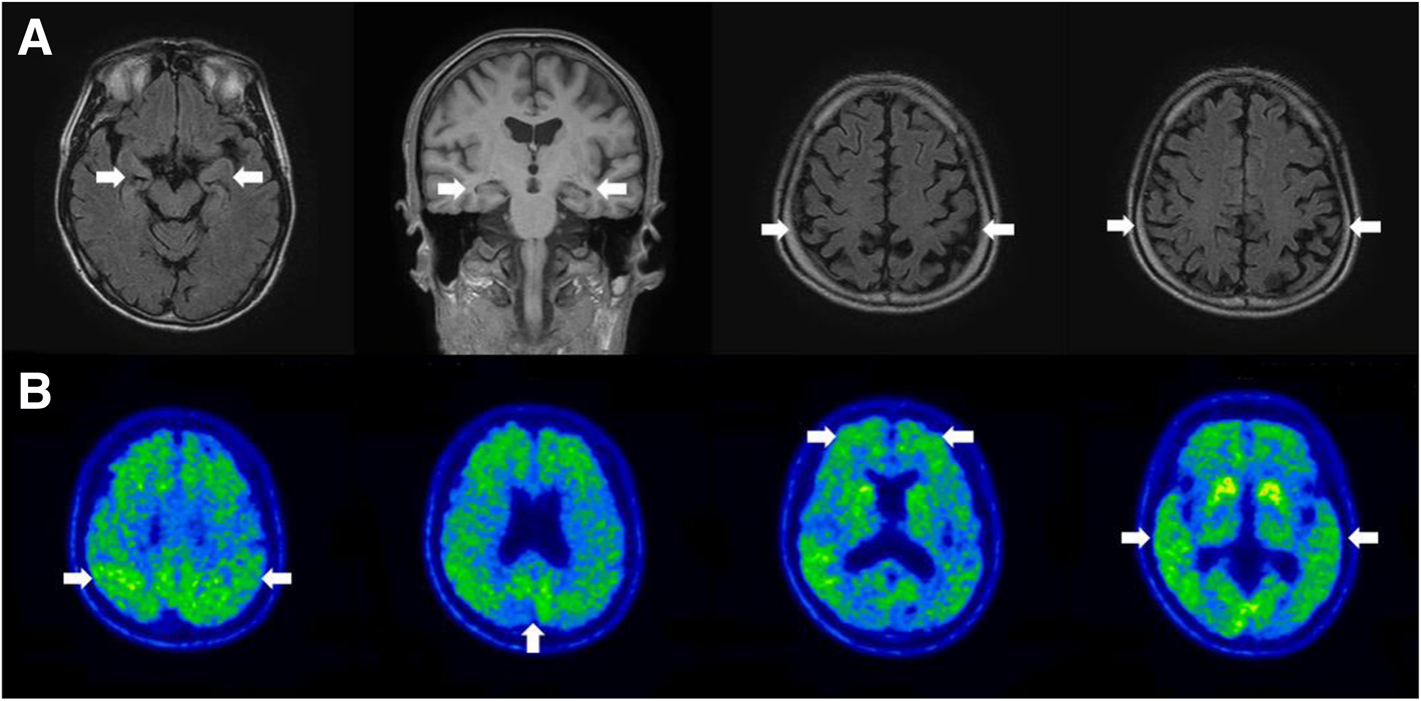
Brain functional and structural neuroimaging data of the proband at diagnosis. a. Axial FLAIR, coronal, and sagittal T1 images of brain MRI, arrows pointing at left-dominant bilateral temporal lobe atrophy. b. FDG-PET, arrows pointing at hypometabolism in left temporal cortex, right anterior temporal cortex and bilateral frontal cortex
